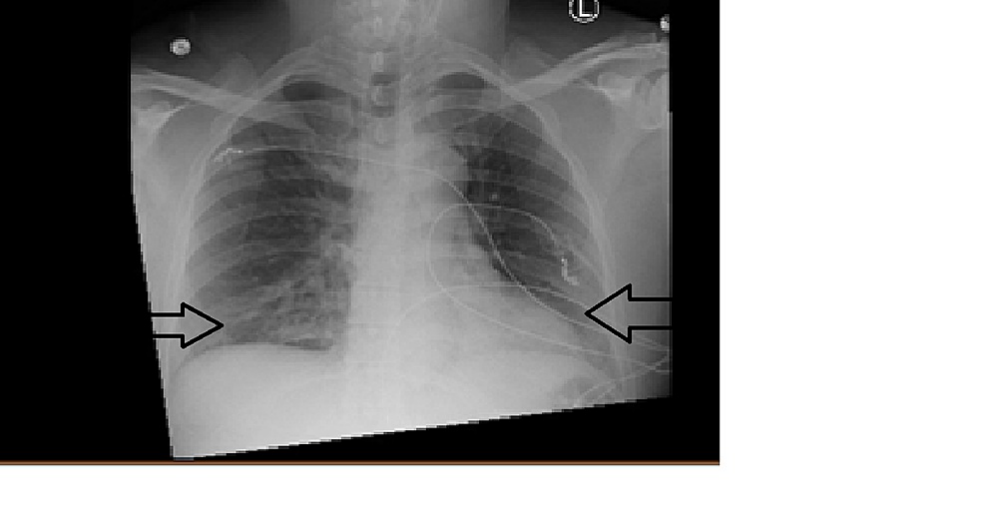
Chest x-ray showing bilateral opacities
